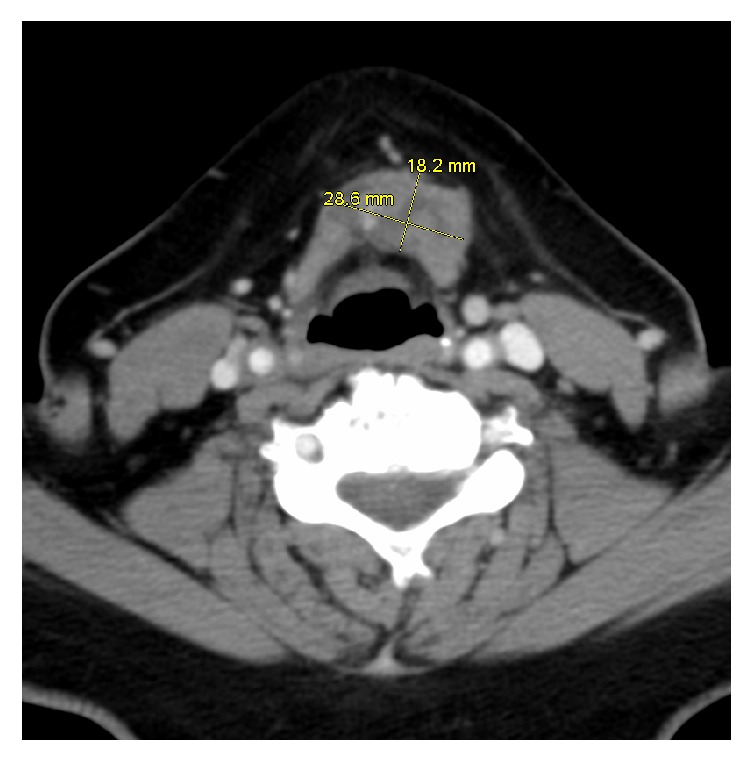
Transverse view of the CT neck that showed presence of a neck mass measuring 28.6 mm × 18.2 mm.
